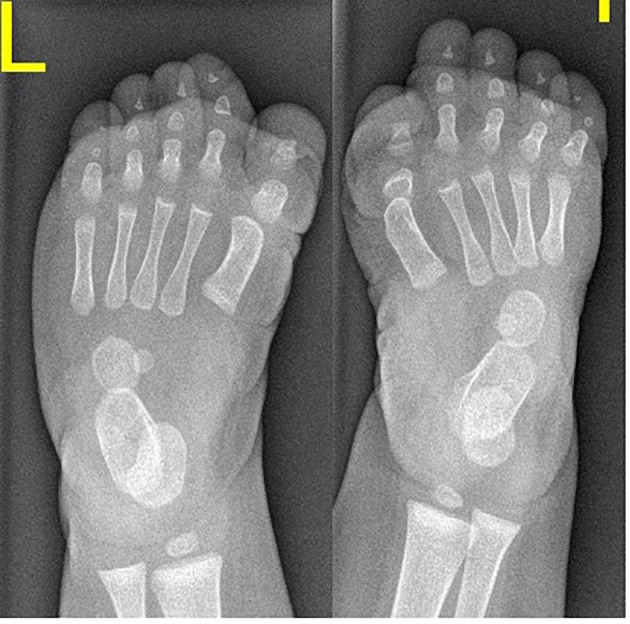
AP radiograph of a 6-month-old girl showed the characteristic shortening and broadening of the first metatarsal which was angulated medially with hypoplasia of the basal phalanx of the first toe - triangular distal phalanx. These features are the earliest in the diagnosis of FOP, making the diagnosis possible before the appearance of soft tissue calcification.
